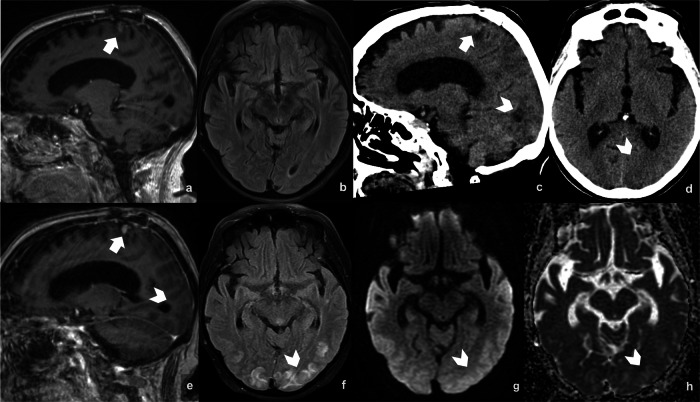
PRES in a patient with NSCLC brain metastasis treated with Pembrolizumab and SRS. Baseline MRI (a, b) shows recurrence of a left precentral metastasis (arrow). Eleven days after treatment, the patient presented with hypertension and altered mental status. CT (c, d) revealed bilateral parieto-occipital hypodensities (arrowheads), suggestive of PRES. MRI (e–h) confirmed bilateral parieto-occipital T1 hypointense areas (e), T2-FLAIR hyperintense regions (f), and facilitated diffusion on DWI/ADC (g, h), consistent with a PRES diagnosis
